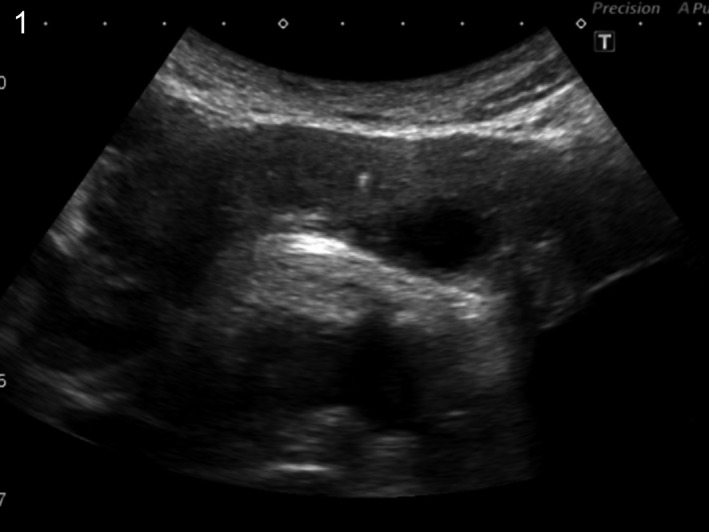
Abdominal ultrasonography showed a spot of high echogenicity in the pancreas indicating calcification secondary to chronic pancreatitis
